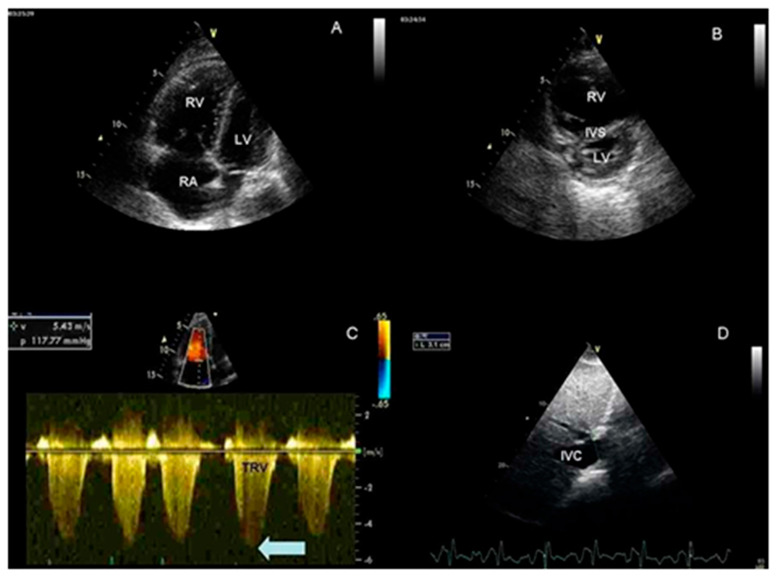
Echocardiography of a patient with idiopathic pulmonary arterial hypertension. (A,B) Apical 4-chamber and parasternal short-axis views, showing severe RV enlargement and pathologic migration of the IVS toward the LV. (C) Doppler tracing of TR, showing severe PH (TRV > 5 m/sec) (arrow). (D) Dilated IVC from the subcostal view. RA = right atrium; RV = right ventricle; LV = left ventricle; IVS = interventricular septum; TR = tricuspid regurgitation; PH = pulmonary hypertension; TRV = tricuspid regurgitation peak velocity; IVC = inferior vena cava. Reprinted/adapted with permission from Ref. [79].
